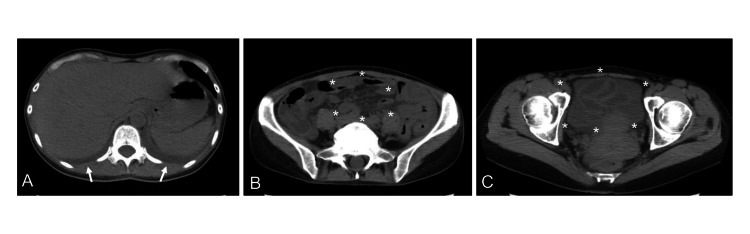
A follow-up abdominal CT scan conducted six days after admission to our hospital. Follow-up abdominal CT scan performed six days after hospital admission, showing pleural effusions (A; arrow), intra-abdominal stranding (B; asterisk), and extensive ascites (C; asterisk).
